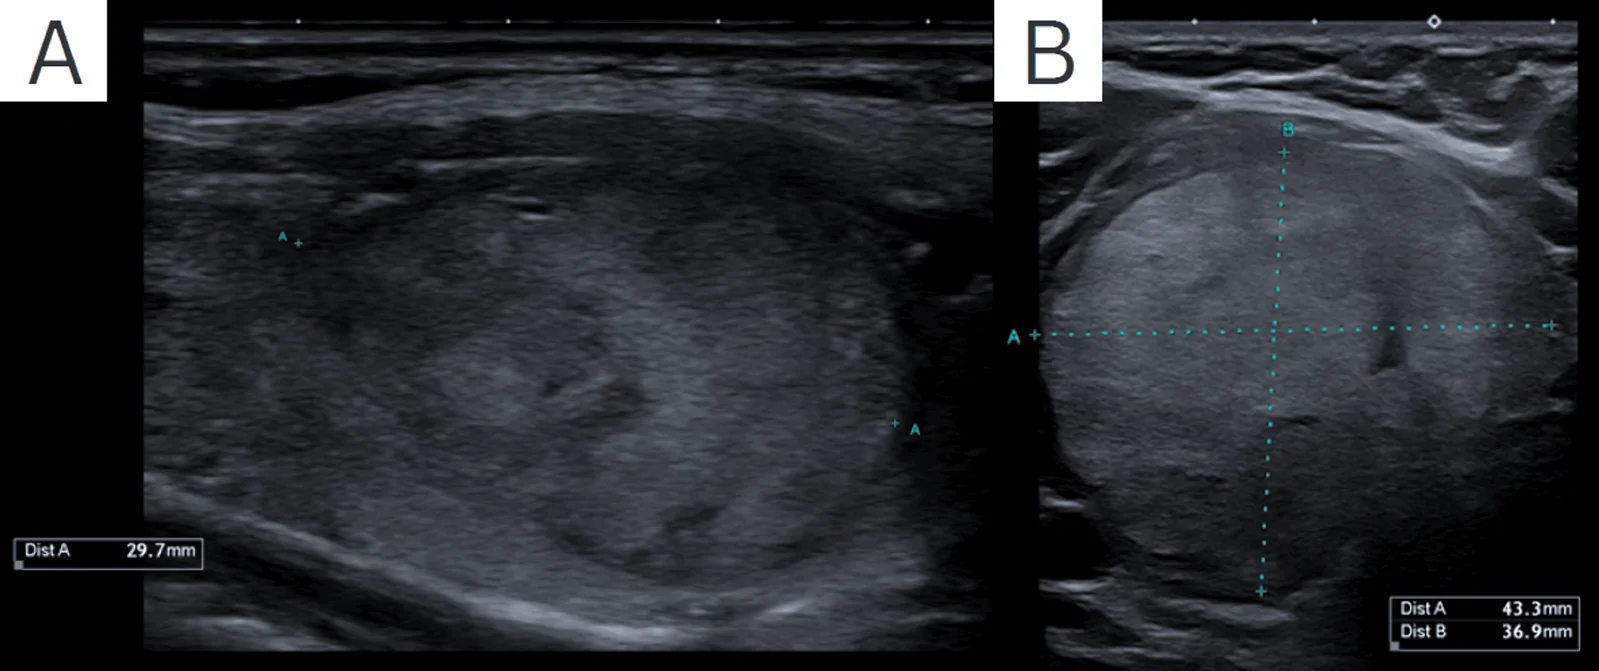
Ultrasound images showing greatest radiological dimension of nodules of first (A) and second (B) cases
